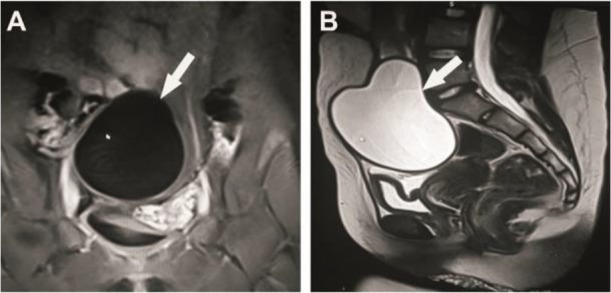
The MR image of the cystic mass (A: T1A, the coronal section pressured by fat, B: T2A, the sagittal section)
